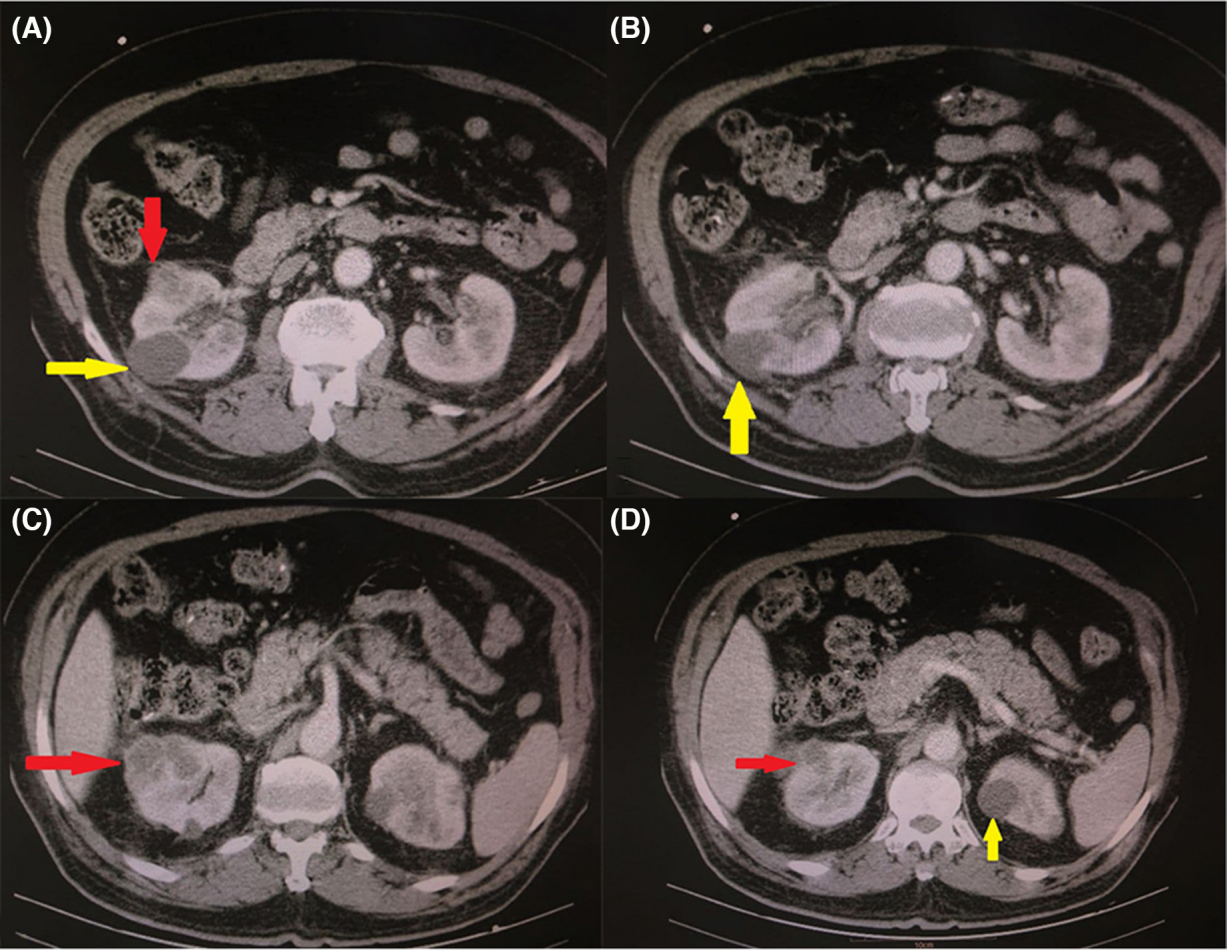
Abdominal CT displays hypodense masses in right kidney (red arrows) and the two simple cysts in both sides (yellow arrows).
